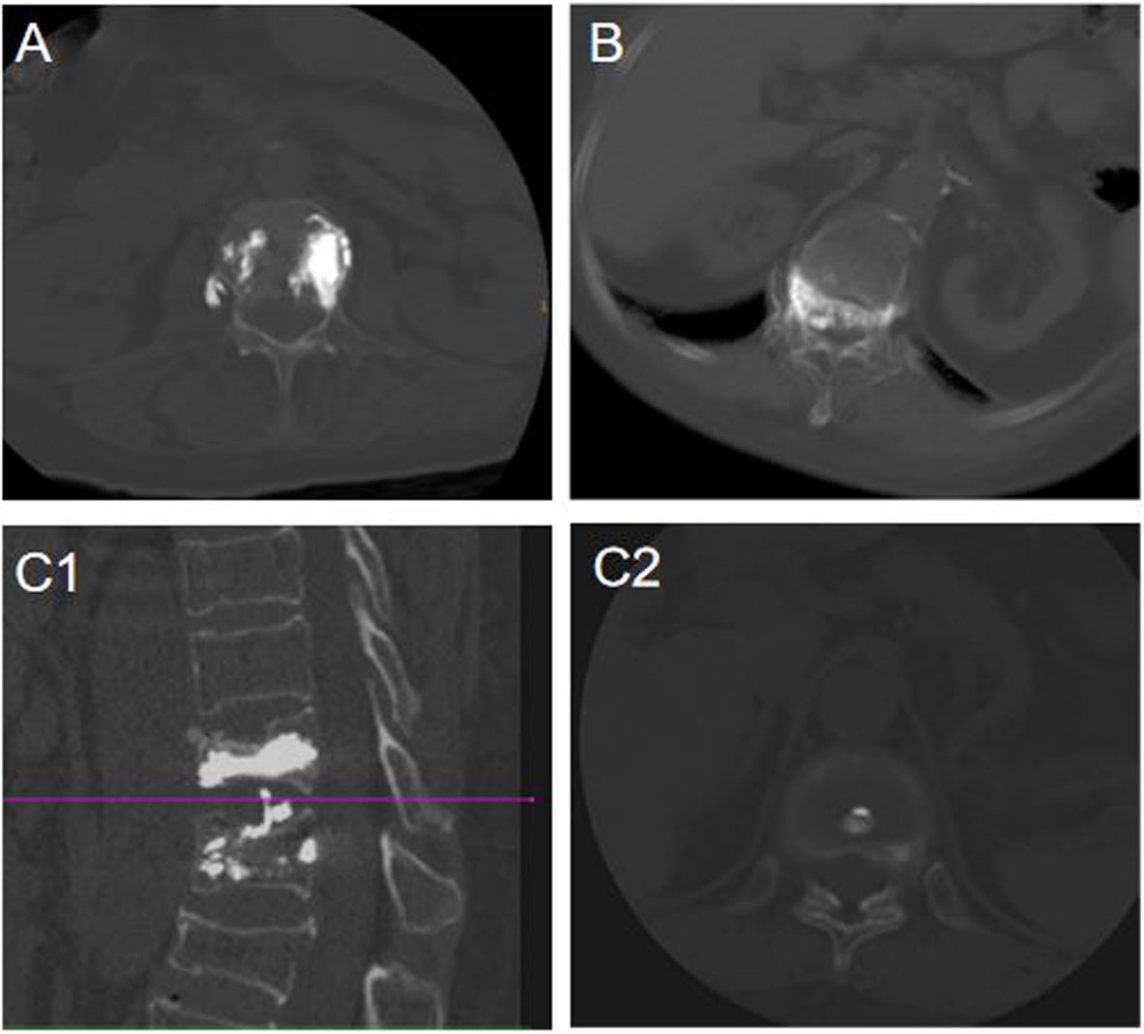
Representative images of cement leakage on a computed tomographic scan. Paravertebral cement leakage in transverse view (A). Intraspinal cement leakage in transverse view (B). Intradiscal cement leakage in sagittal and transverse view (C1, C2)
